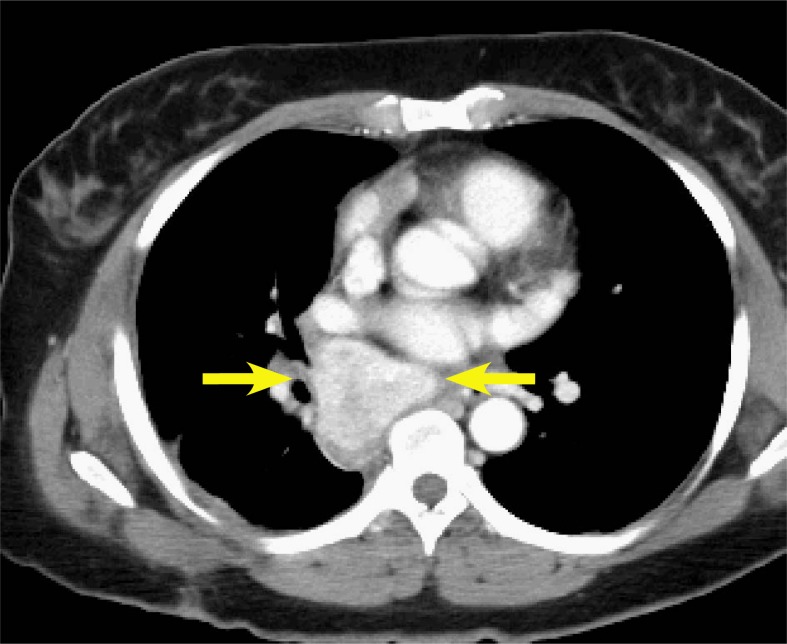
CT of patient at initial presentation with a mass noted in the posterior right mediastinum.
